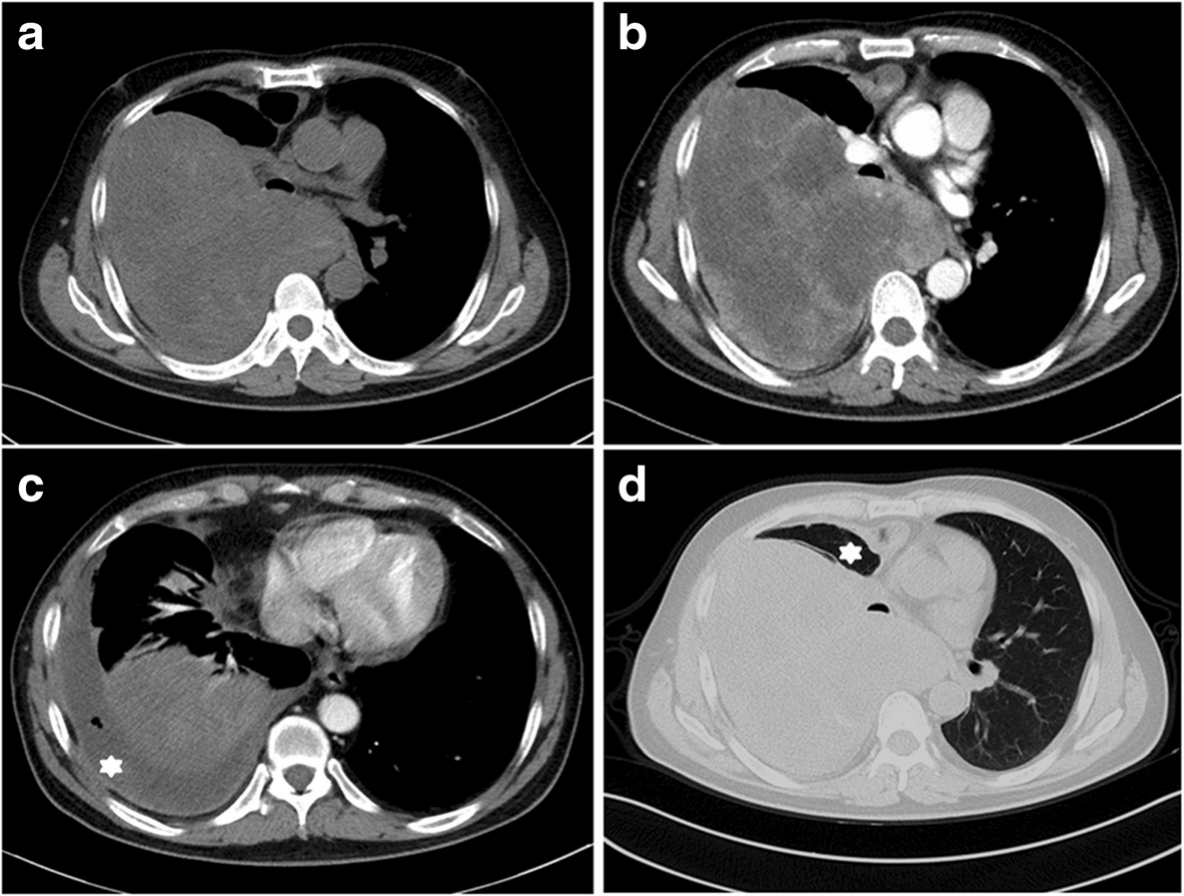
Primary synovial sarcoma of the lung in a 47-year-old male with chest pain and dyspnea. a Non-contrast CT (mediastinum window) scan demonstrating a large mass of the right lung, contiguous with the right lateral pleura and the mediastinum, note the mediastinal shift. b Contrast-enhanced CT scan (mediastinum window) showing the heterogeneous enhancement mass with predominantly cystic areas and a massive pleural effusion (*white asterisk*, c). d Contrast-enhanced CT scan (lung window) revealing obvious atelectasis on the right (*white asterisk*)
